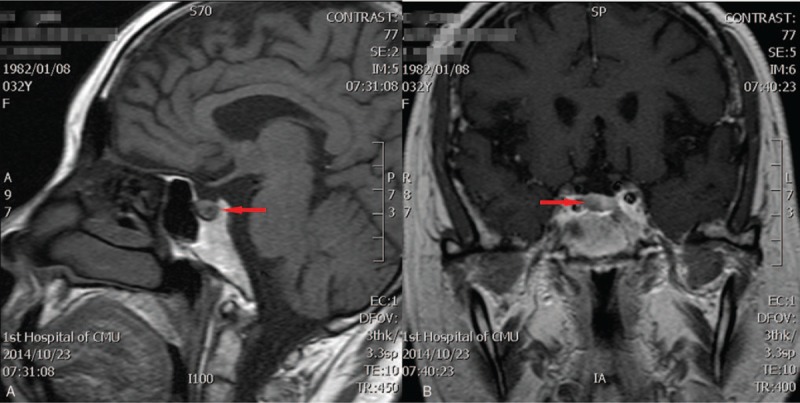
(A) Magnetic resonance imaging (MRI) scan showing a neoplasm (red arrow) that is slightly hypointense on T1-weighted sagittal image. (B) Preoperative coronal MR images.
